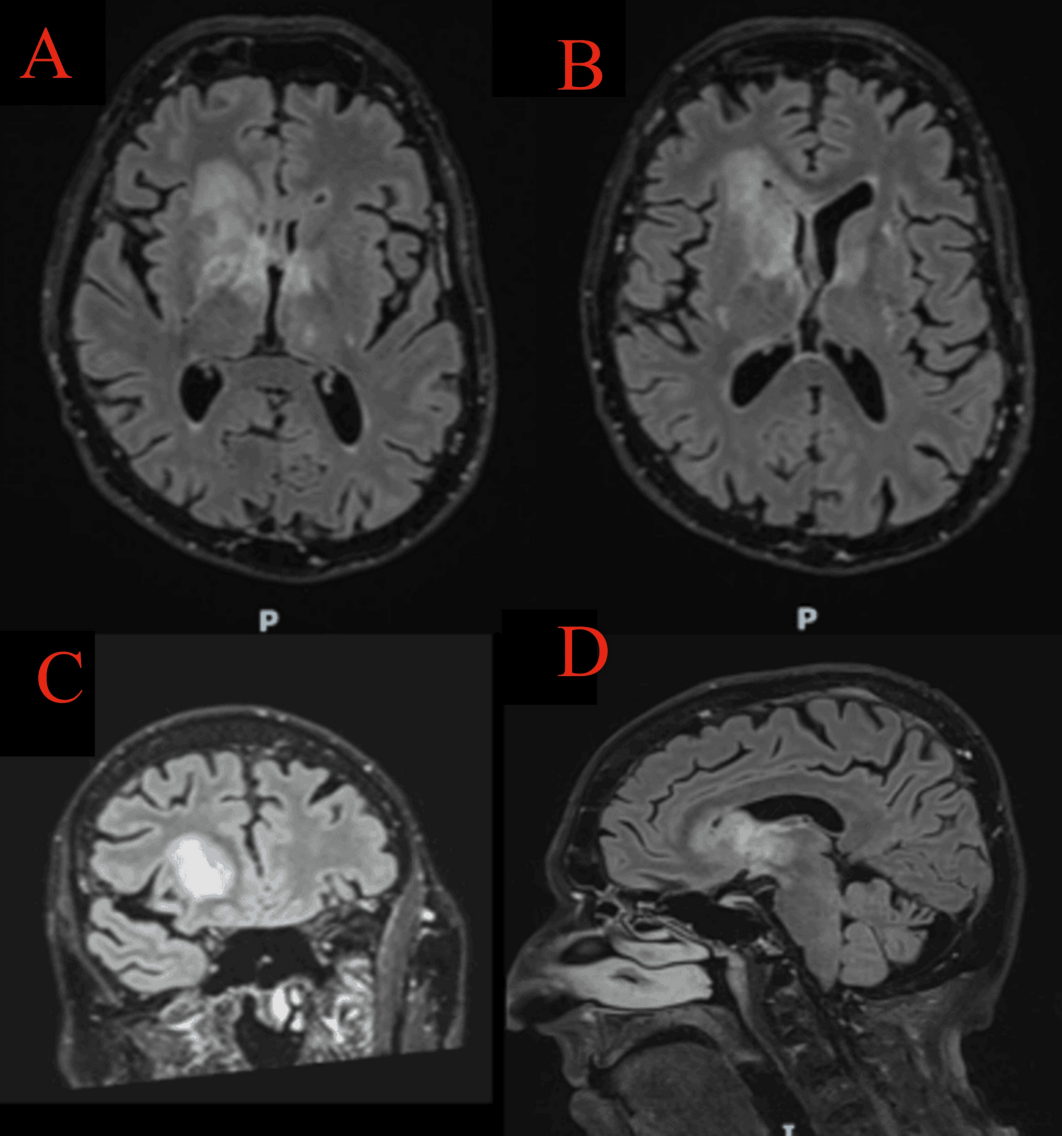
Brain MRI (FLAIR) Brain MRI scans of FLAIR demonstrated multiple high-intensity regions. Axial views (A, B) reveal hyperintensities in the right corpus striatum, extending to the frontal periventricular region, anterior thalamus, corticospinal tract, and splenium of the corpus callosum. Coronal imaging (C) highlights further involvement of the left anterior thalamus, raising concern for contralateral disease. The sagittal view (D) delineates the extent of corpus callosal involvement. There is no evidence of hemorrhage, hydrocephalus, or acute infarction. These findings suggest differentials of high-grade glioma or primary central nervous system lymphoma (PCNSL). FLAIR: fluid-attenuated inversion recovery
